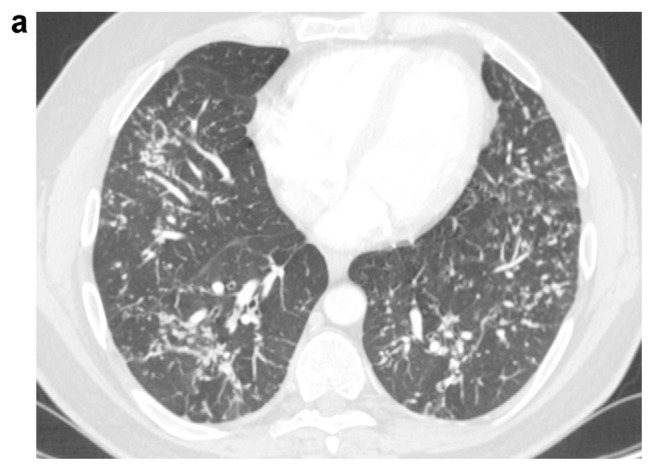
CT scan lung windows (1.25 mm collimation) of lower lung lobes: nodular thickening of contiguous interlobular septa and subpleural tiny nodules in posterior and lateral basilar segments of both lower lobes. Absence of distortion and/or traction bronchiolectasis.
